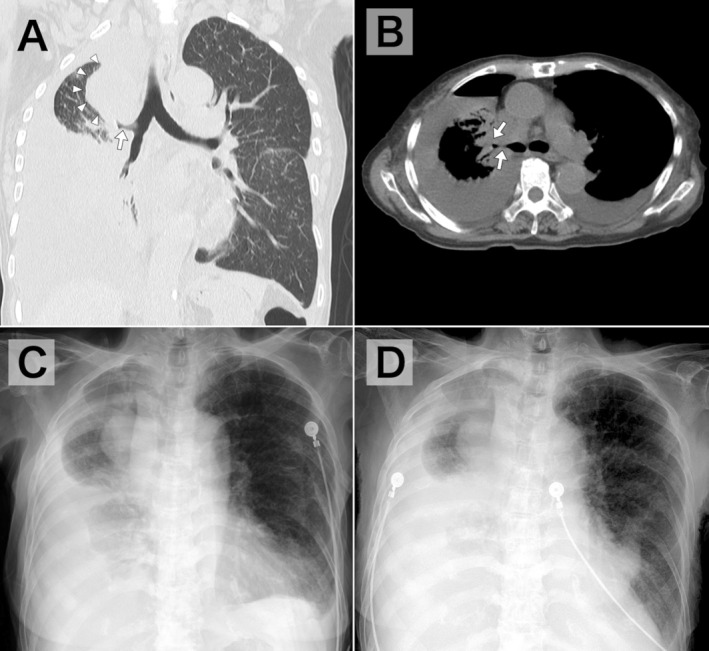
(A) Computed tomography obtained after thoracentesis, revealing a right hilar tumor (white arrowheads) obstructing the right bronchus (white arrow). (B) Axial mediastinal‐window view demonstrating obstruction of the right bronchus (white arrows). (C, D) Follow‐up chest radiographs (anteroposterior view, seated position) obtained 2 and 5 days after thoracentesis, respectively, showing reaccumulation of pleural effusion without lung shape change.
